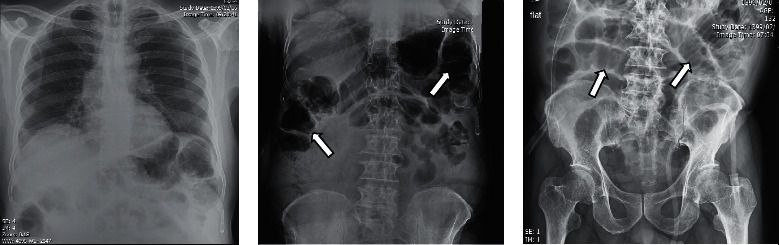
The patient's chest X-ray: (a) no significant finding in chest X-ray; (b) colon is visible; (c) dilated small bowel loops.
